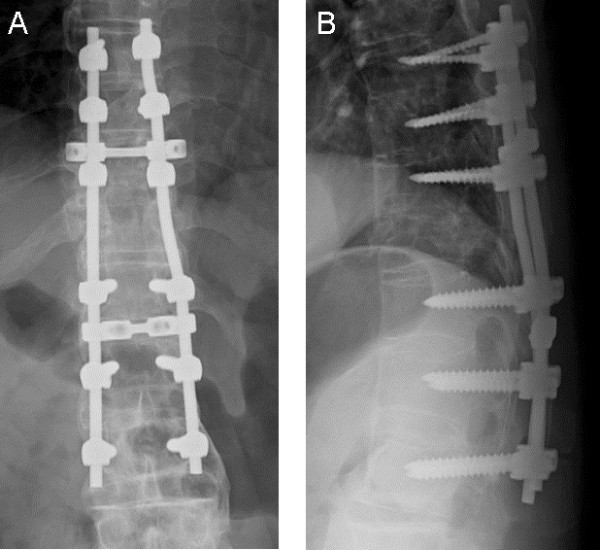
Anteroposterior (A) and lateral (B) radiographs taken a week after surgery of a patient with Parkinson’s disease with a thoracic spine fracture in a severely ankylosed spine. Pedicle screws were inserted from T8 to L2 levels, except in the fractured T11 vertebral body.
